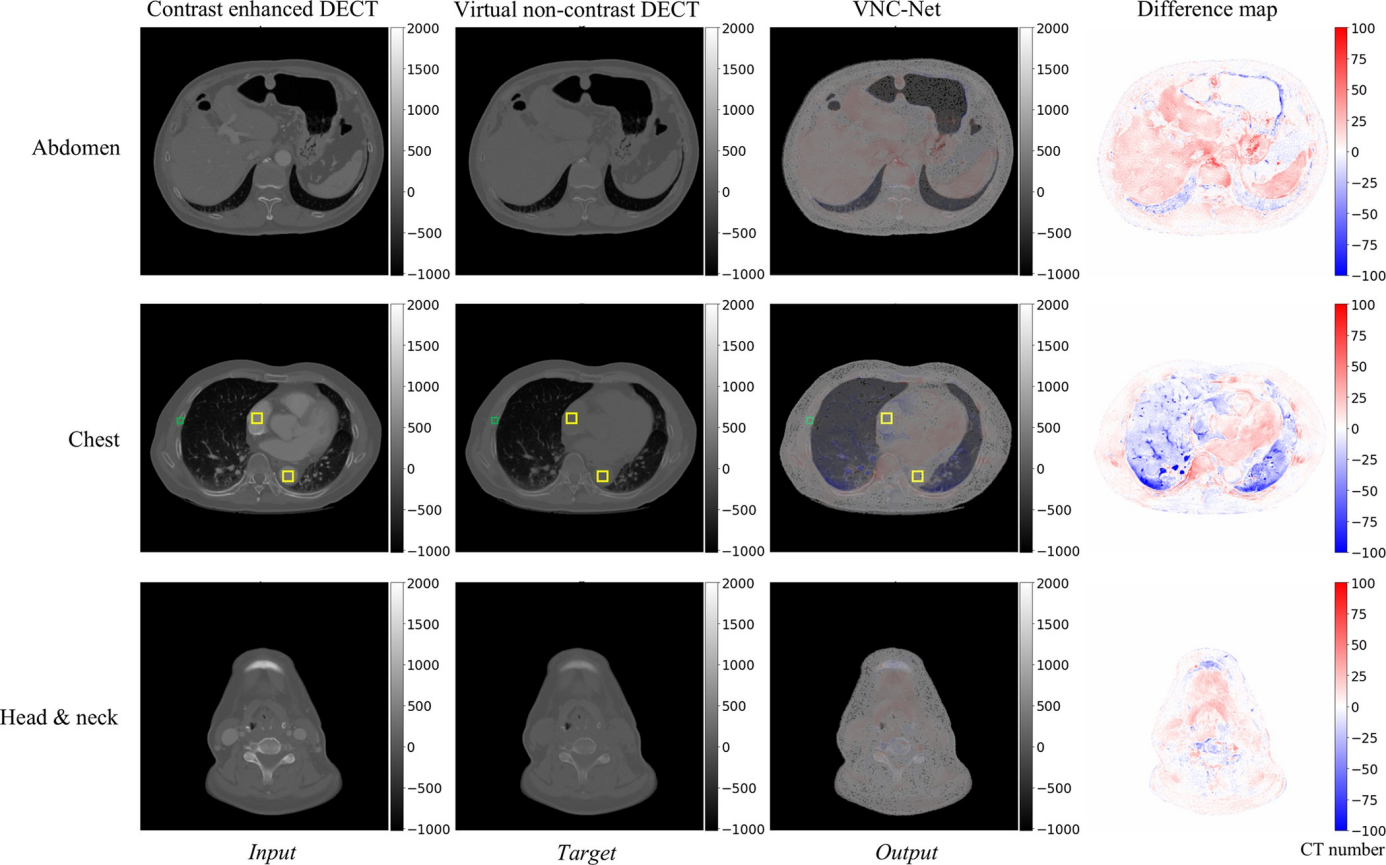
VNC-Net model results for DECT dataset. Three samples from the test set are visualized. The rightmost column represents the difference map between the target and model output, and it is overlaid on the model output in the third column to highlight where the anatomy differs from the target. The images in the middle row have the right atrium (marked by the upper left yellow box of each image), the descending aorta (marked by the lower right yellow box of each image), and the rib (marked by the green box of each image) as the ROIs. DECT, dual-energy computed tomography; ROI, region of interest.
